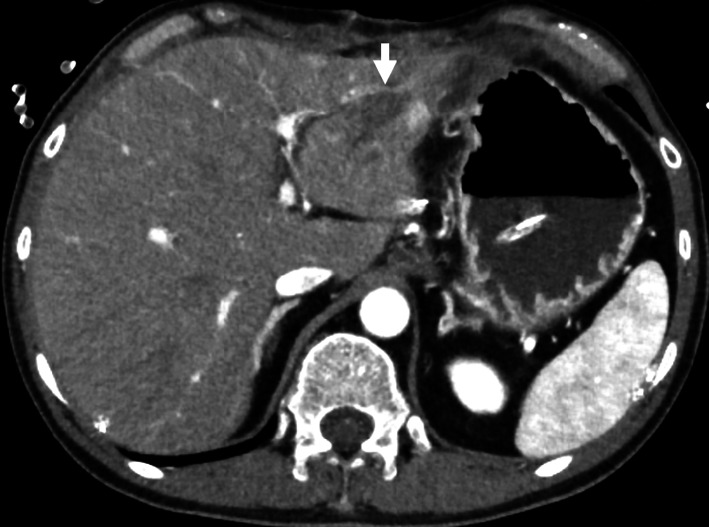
Computed tomography on postoperative day 12 showed a small amount of intrahepatic fluid in a penetrating wound in the lateral segment of the liver (the white arrow). There was no hepatic pseudoaneurysm
